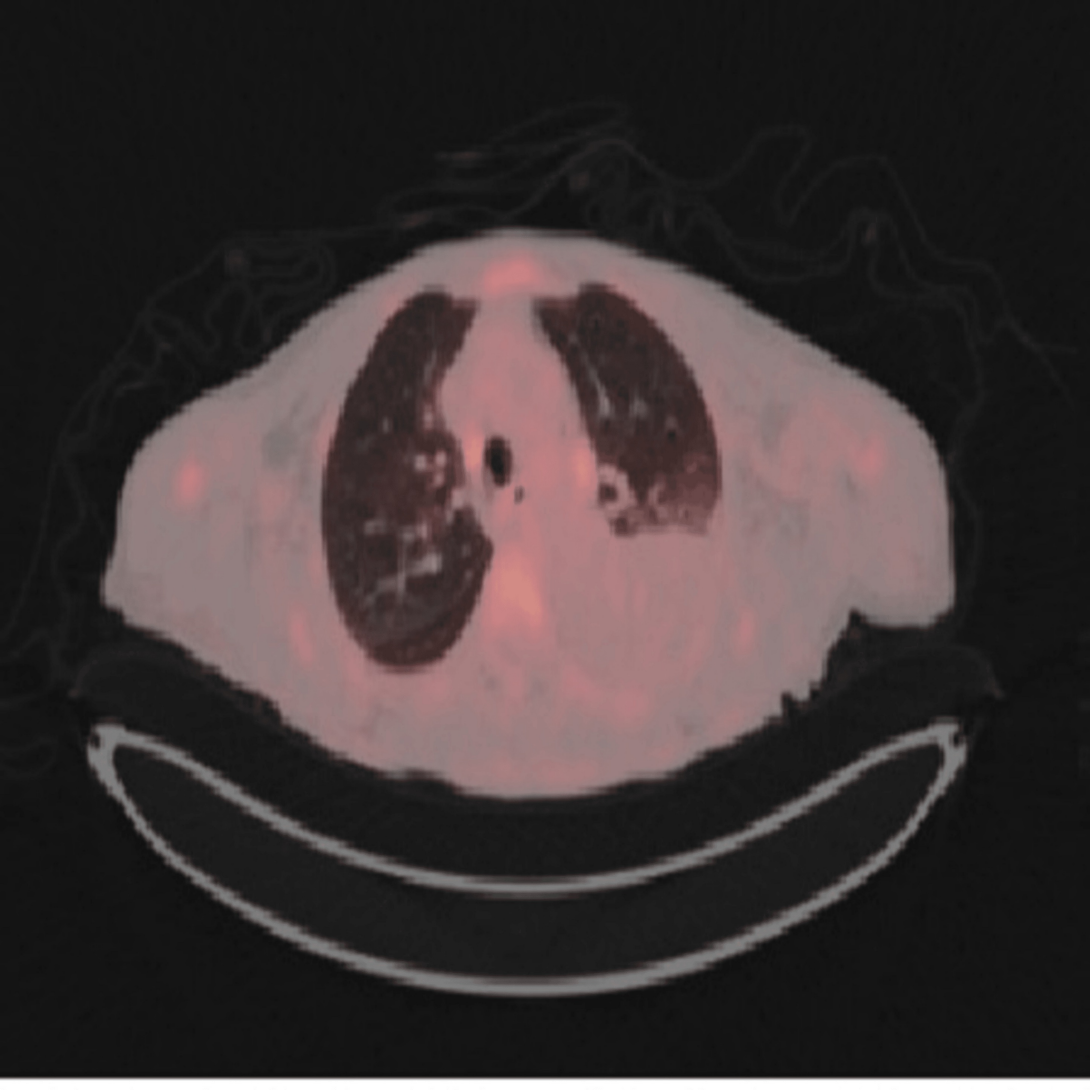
PET scan-thoracic window showing FDG-avid mediastinal lymph nodes. FDG: fluorodeoxyglucose.
